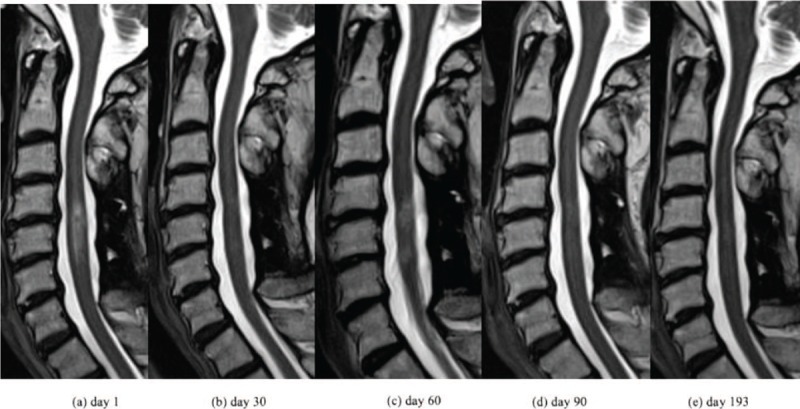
Cervical spinal magnetic resonance imaging (short-tau inversion recovery sequence). Day 1, (b) Day 30, (c) Day 60, (d) Day 90, (e) Day 193.
